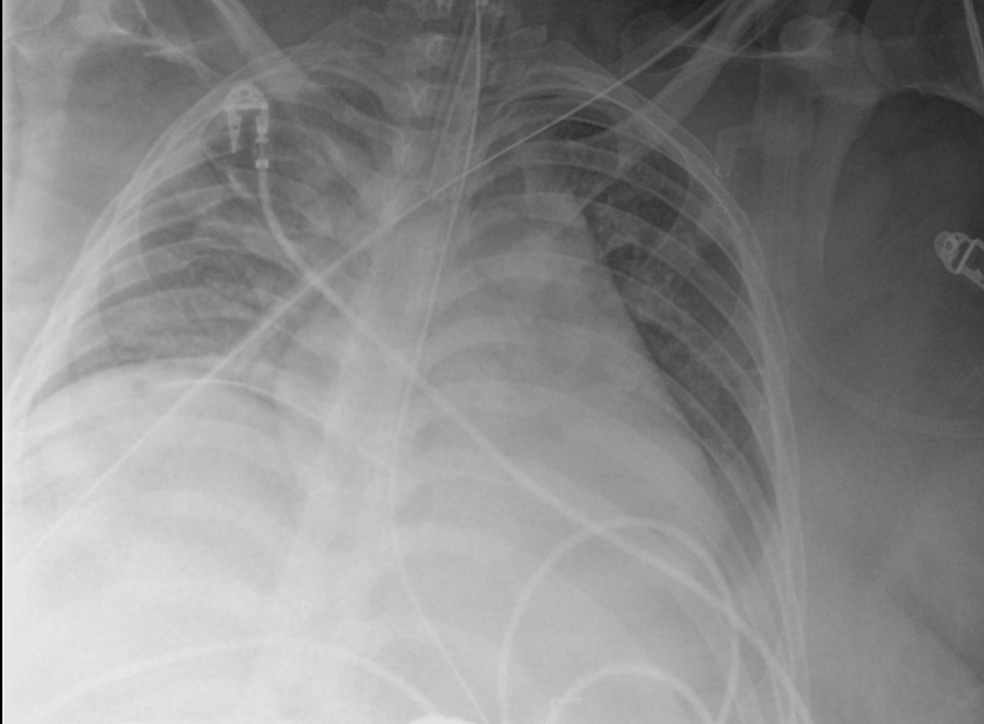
Portable chest X-ray 36 hours after tube thoracostomy placement shows near total resolution of the right-sided subcutaneous emphysema.
